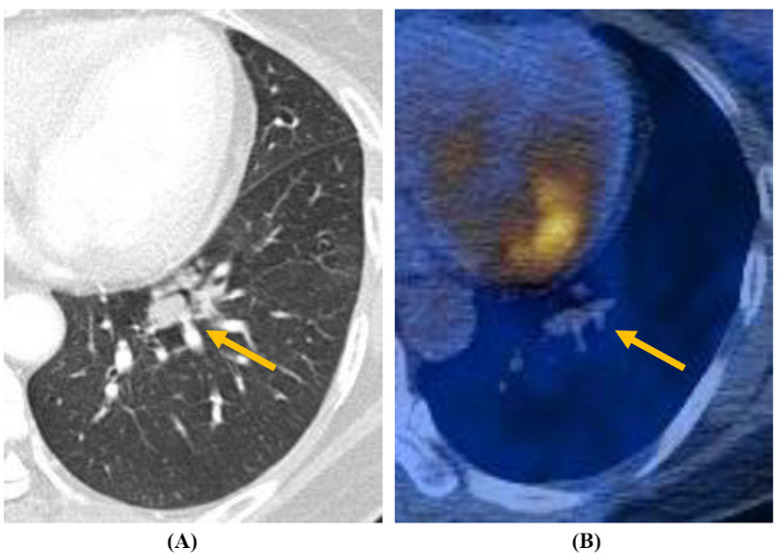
Cavitary *Aspergillus* nodule (arrowhead) from a 62-year-old woman. (A) Chest CT revealed a 23 mm round nodule in the left lower lobe. (B) PET/CT uptake showed a SUV_max_ value of 2.0.
